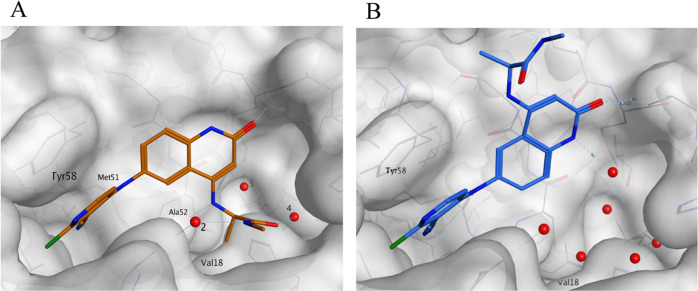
(A) X-ray structure of pocket-facing enantiomer (*R*)-ethyl amide 12b (PDB: 7OKI, resolution 1.61Å). (B) X-ray structure
